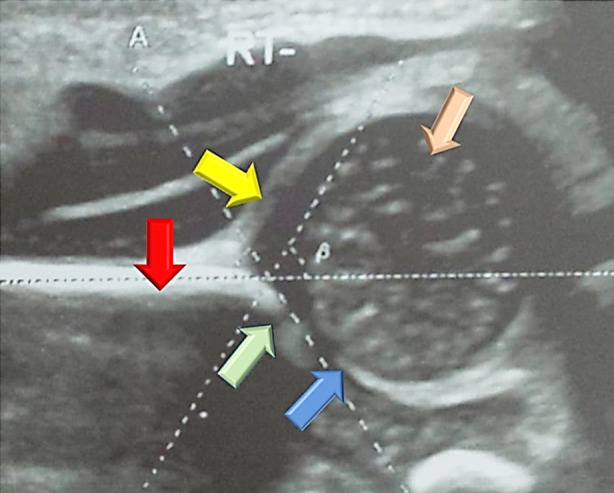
The Anatomical Landmarks used to measure Hip Angles on Graf’s Method Ultrasound indicated by arrows.
